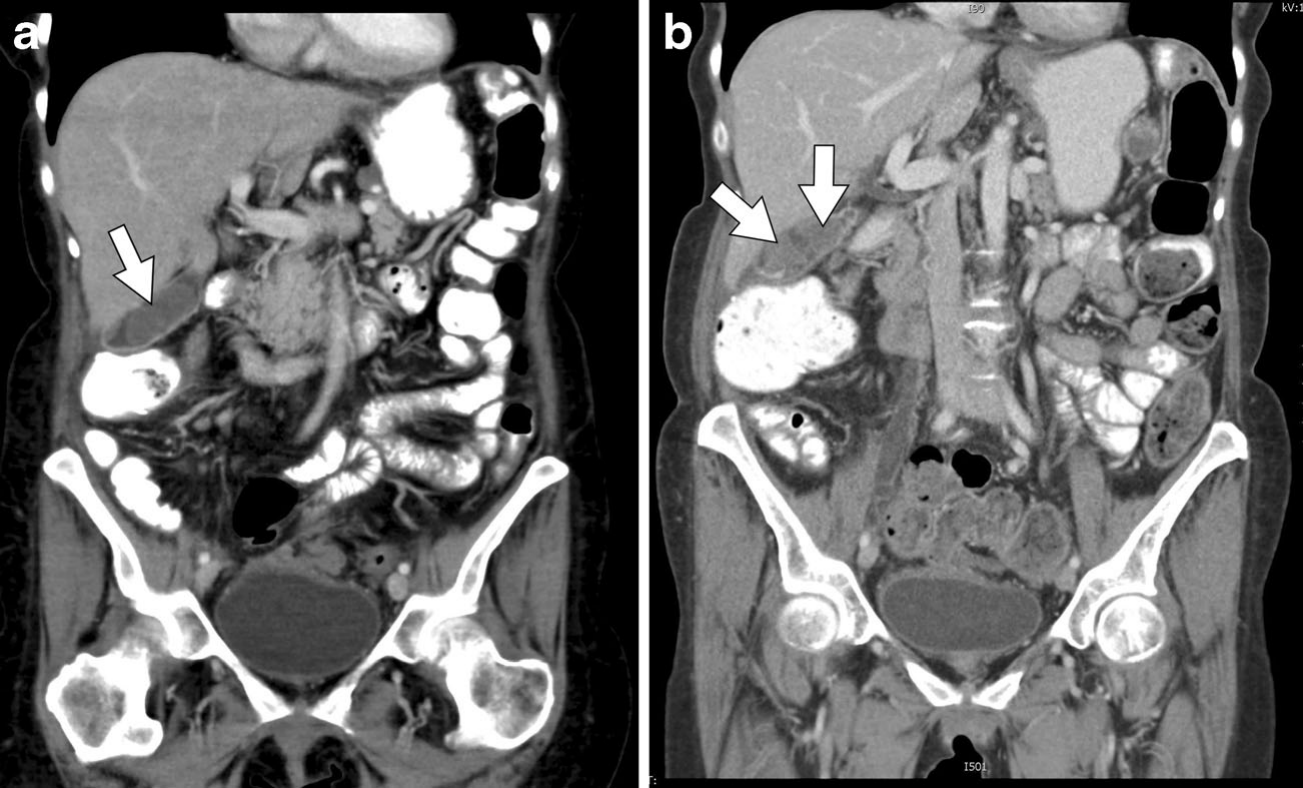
A 60-year-old female with recurrent ovarian cancer treated with a multi-drug chemotherapy regimen, which included gemcitabine. a Coronal contrast-enhanced CT following chemotherapy shows initial presentation of tumefactive sludge (*arrow*) within the gall bladder lumen. b Repeat coronal contrast-enhanced CT 6 months later shows progression of tumefactive sludge (*arrows*) and enhancing mildly thickened gallbladder wall changes, consistent with biliary stasis
